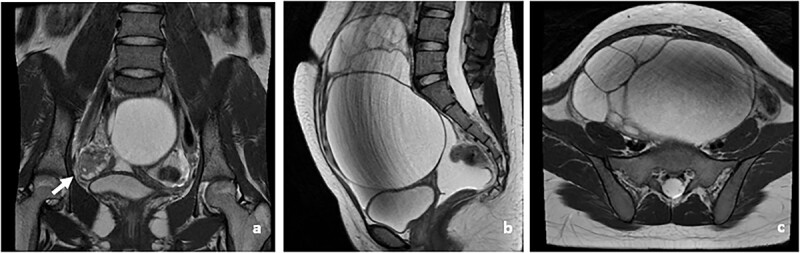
Patient MRI (a). Pelvic MRI showing normal right ovary (white arrow) (b). Sagital view of the left ovarian mass (c). Transverse view of the left ovarian mass.
